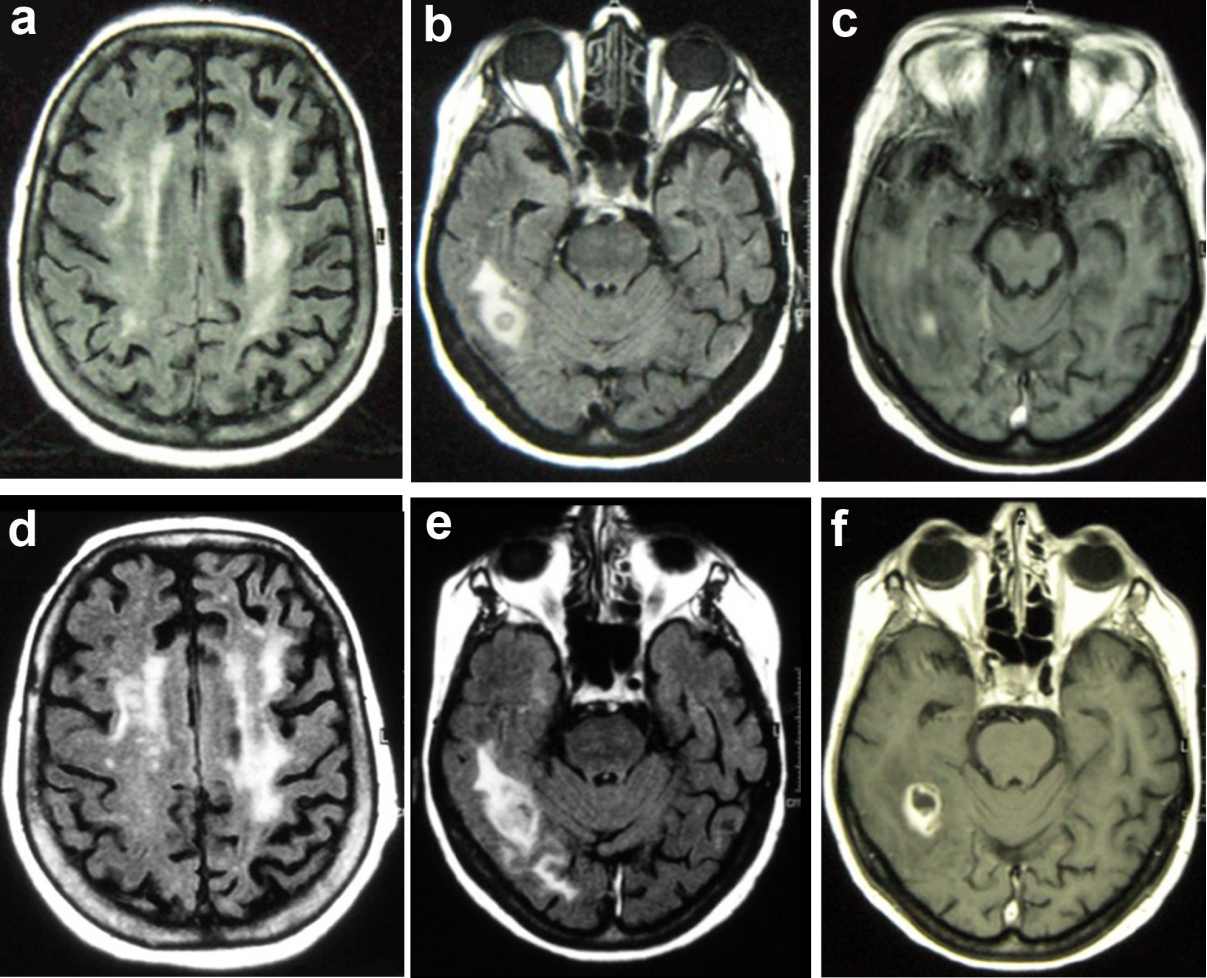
Central nervous system (CNS) immune reconstitution white matter lesions and *R. equi *brain abscesses by Magnetic Resonance Imaging (MRI) (case report 1). a-c. Brain lesions at the onset of focal neurological symptoms. a. Axial FLAIR brain sequence showing non specific asymmetric bilateral hyperintensity in the subcortical region, expression of immune recconstitution inflammatory reaction. b. Axial FLAIR sequence showing abscessual lesion, surrounded by oedema, in the right temporal region. c. Gadolinium (Gd)-T1 sequence shows the presence of nodular enhancement of the right temporal lesion. d-f. Evolution of brain lesions 1 month after onset of symptoms. d. Axial FLAIR sequence shows the persistence of the non specific white matter hyperintensity; e. Axial FLAIR sequence shows an increase of lesion size and oedema of the right temporal lesion; f. Gd-T1 sequence shows the evolution of contrast enhancement, now presenting as ring enhancement, typical of an abscessual lesion.
